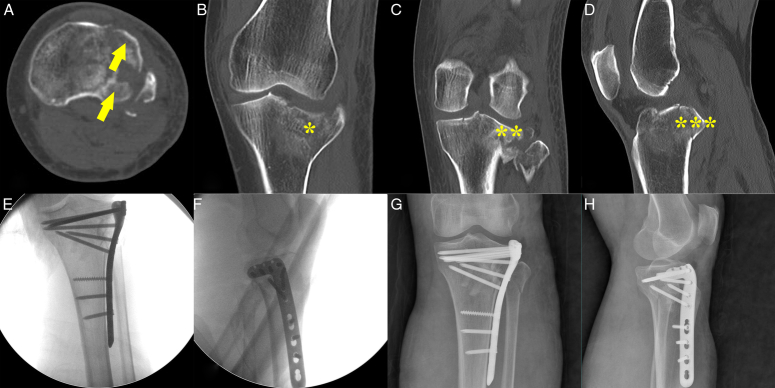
The therapeutic protocol for PL+AL fractures. (A–D) The preoperative CT scan shows the fractures affecting both the AL and PL quadrants, with instability in the posterior slope. * AL fractures.  PL fractures. * Posterior slope instability. (E,F) Intraoperative X-rays reveal that the articular surface of both quadrants is adequately supported by a lateral buttress-rafting plate. (G,H) Postoperative X-rays demonstrate that an excellent reduction effect has been maintained. AL, anterolateral; PL, posterolateral..
